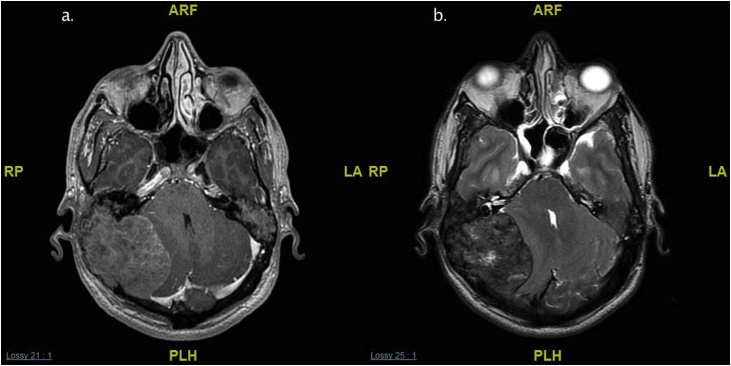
a. T1 weighted image with IV contrast. b. T2 weighted image. MRI showed bilateral heterogenous enhancement of extra-axial posterior fossa lesions originating from the mastoid cavity and petrous bones.
